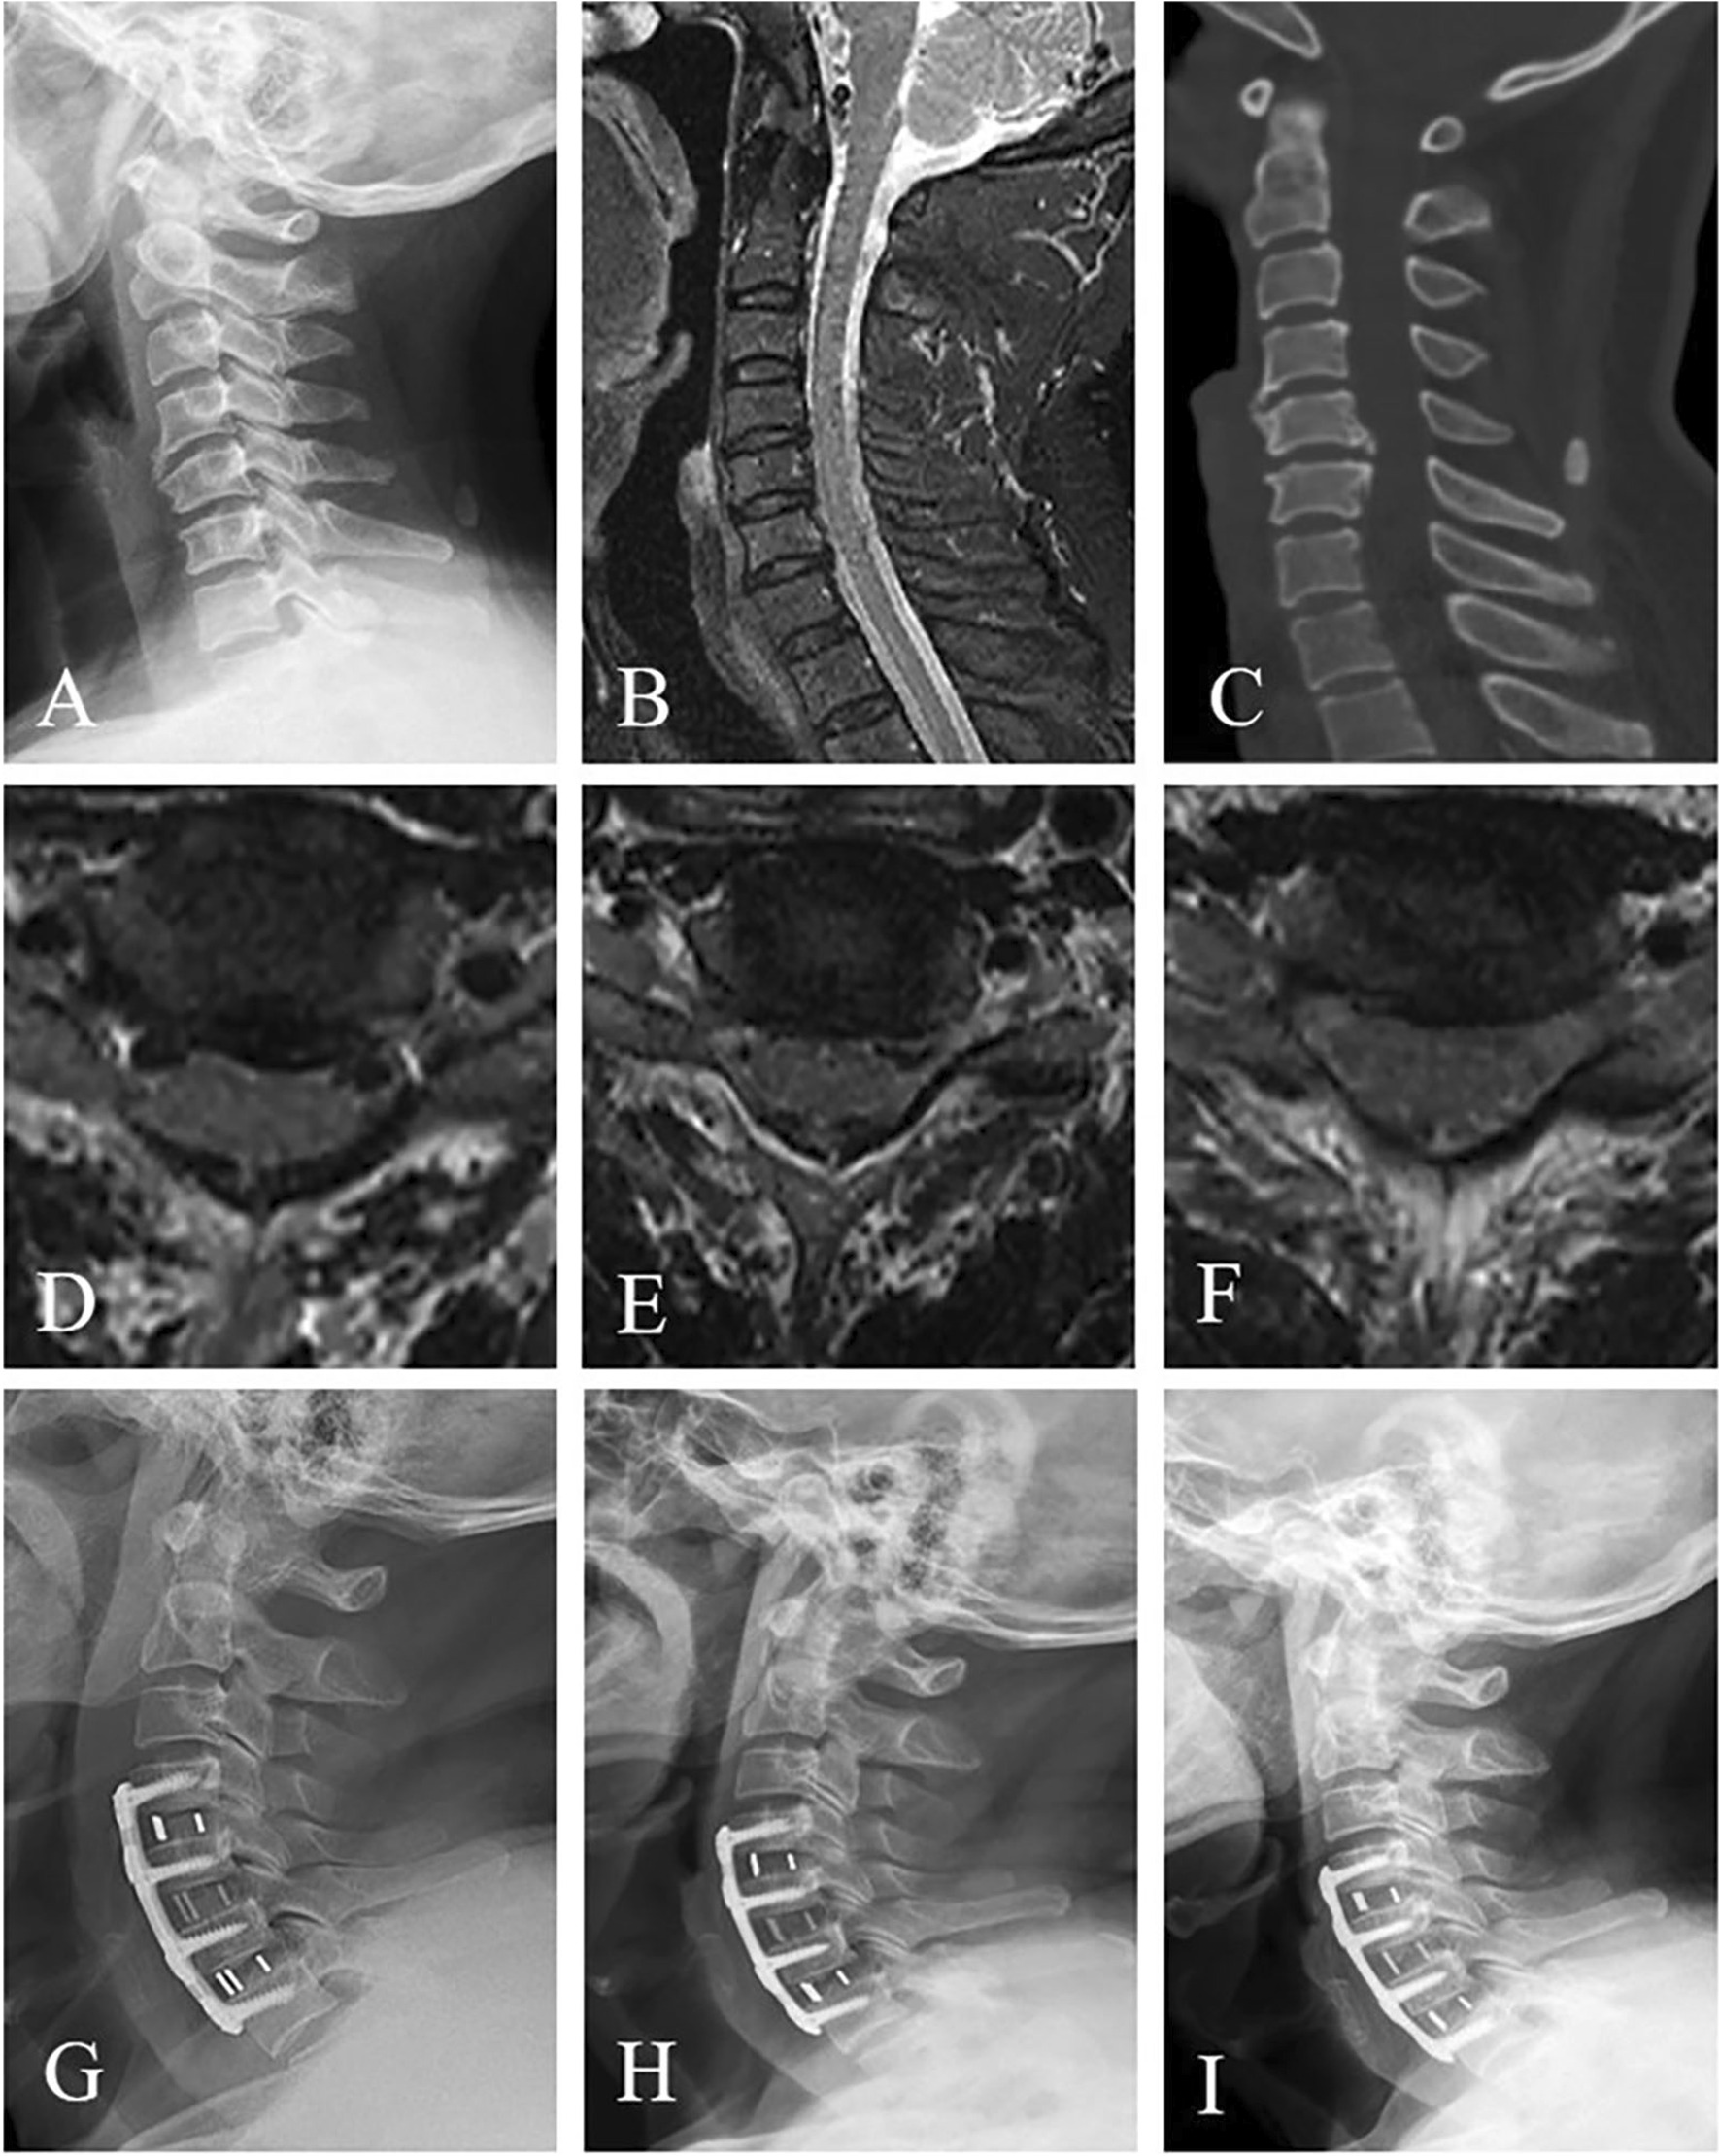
A 63-year-old man who had experienced numbness in both hands and felt stepping on cotton in lower limbs for 3 years was diagnosed as cervical spondylotic myelopathy. A Preoperative cervical lateral X-ray plain radiograph showed the alignment of cervical spine was straight and osteophyte emerged at the anterior edge of the vertebral body. B, D, E, F Cross-sectional and sagittal T2-weighted MRI showed degenerative disc protrusion and spinal cord compression at C4–C5, C5–C6, and C6–C7, respectively. C Sagittal CT reconstruction showed that osteophyte compression was found at C5–6 level. The patient underwent three-level ACDF with anterior cage-with-plate. G The X-ray examination showed that the alignment of cervical spine improved significantly two days postoperatively. H X-ray film which was taken five months postoperatively identified that the alignment of cervical spine was well maintained, and the positions of cages were good. I At fourteen-month follow-up, the lordosis of the cervical spine and the positions of cages were still remained
